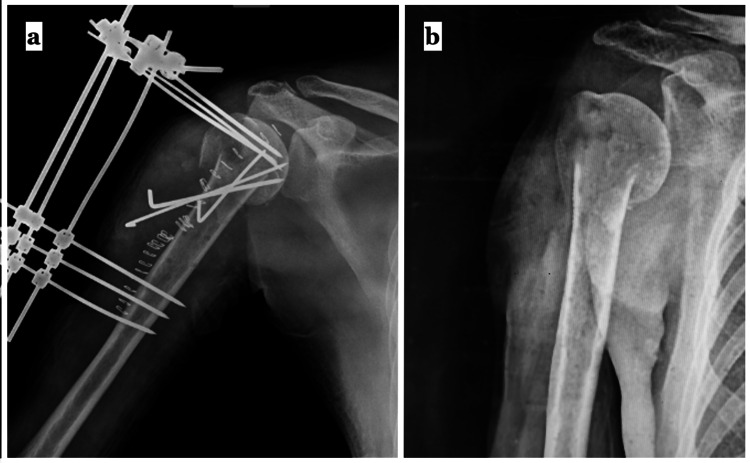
(a) A simple pin-based external fixator was used to salvage the implant failure following the seizure episode. (b) Follow-up radiograph at two months after the second surgery.
